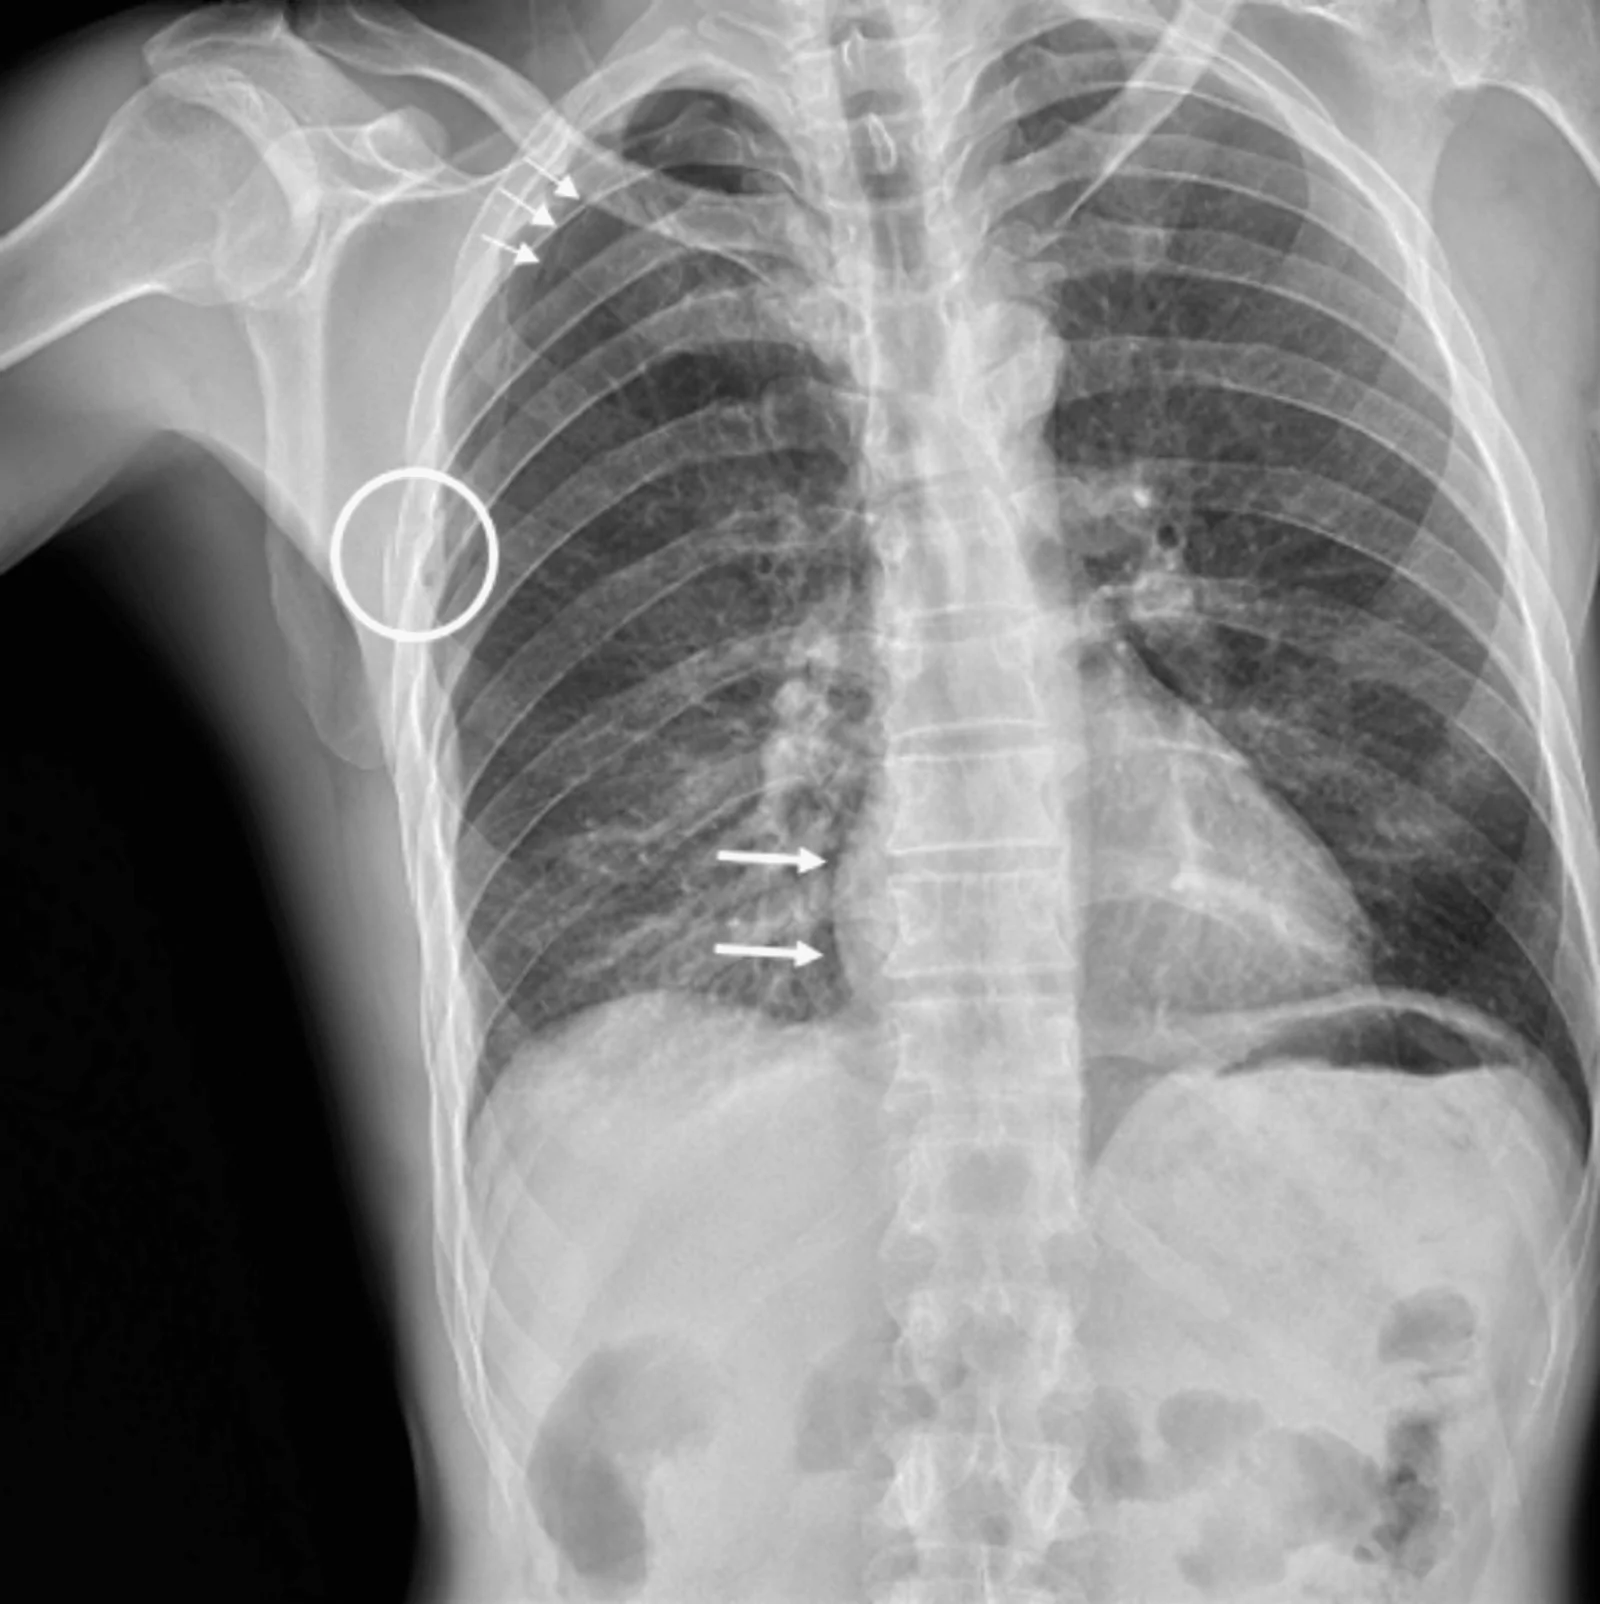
Initial anteroposterior (AP) chest radiograph obtained on the day of trauma. AP chest radiograph demonstrating a right-sided apical pneumothorax (upper white arrows) with no signs of hemothorax. The white circle highlights one of several right-sided rib fractures. Leftward displacement of the cardiac border indicates a mediastinal shift (lower white arrows).
